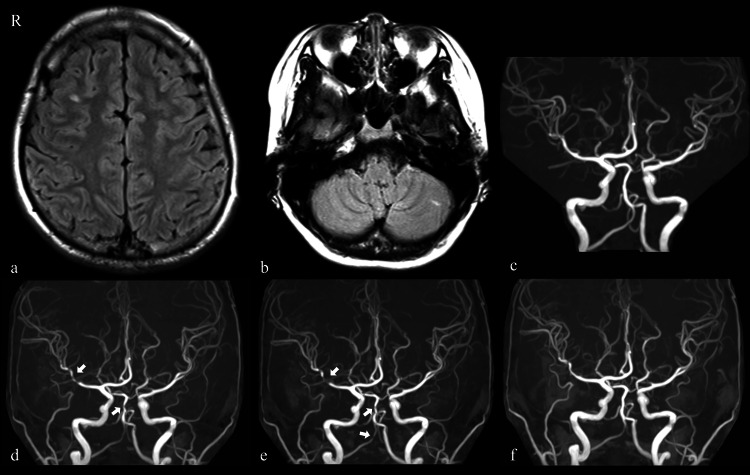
Imaging findings of the patient a-c: MRI of the head revealed multiple cerebral infarctions in the right frontal lobe and left cerebellar hemisphere, with no evidence of vascular stenosis. d: MRA performed on day 34 showing stenosis of the right MCA and BA (arrows). e: By day 47, stenosis of the MCA and BA had progressed, and new stenosis was observed in the right posterior cerebral and right vertebral arteries (arrows). f: The MRA findings normalized by day 52. BA: Basilar artery; MCA: Middle cerebral artery; MRA: Magnetic resonance angiography
